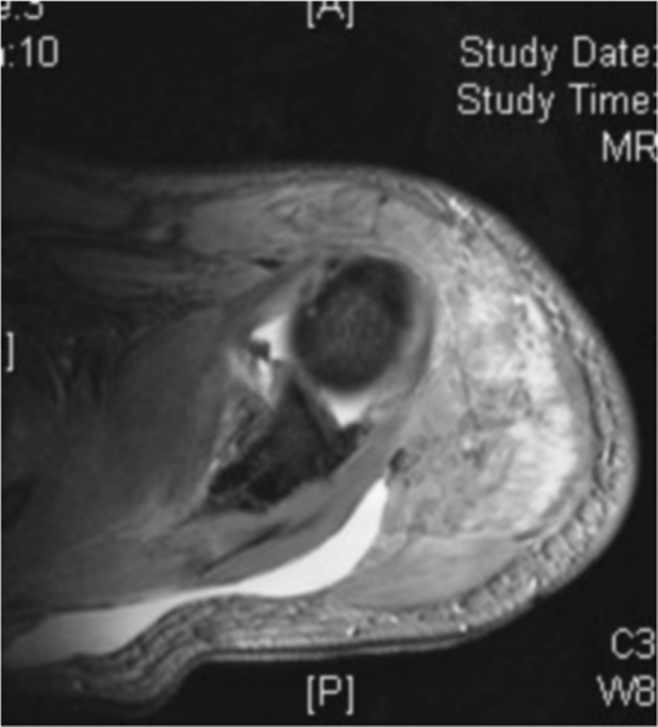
Saggital section of magnetic resonance imaging revealing labral and bicipital tear.
